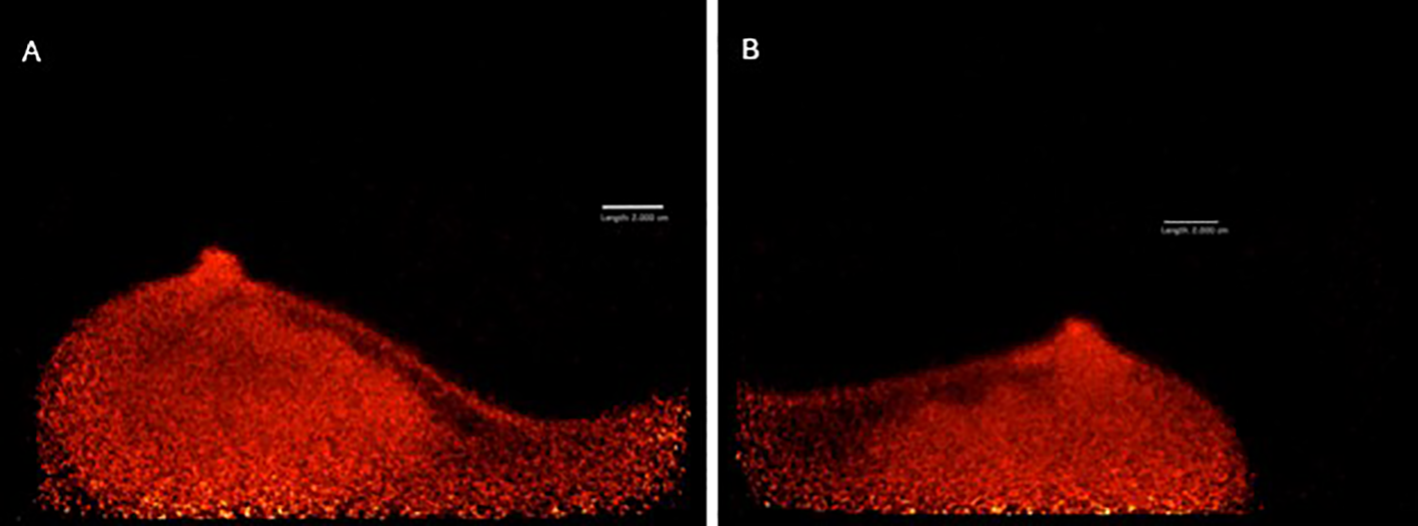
MLO views of left (A) and right (B) breast acquired with Radialis PET Imager showing no regions of focal ^18^F-FDG uptake.
